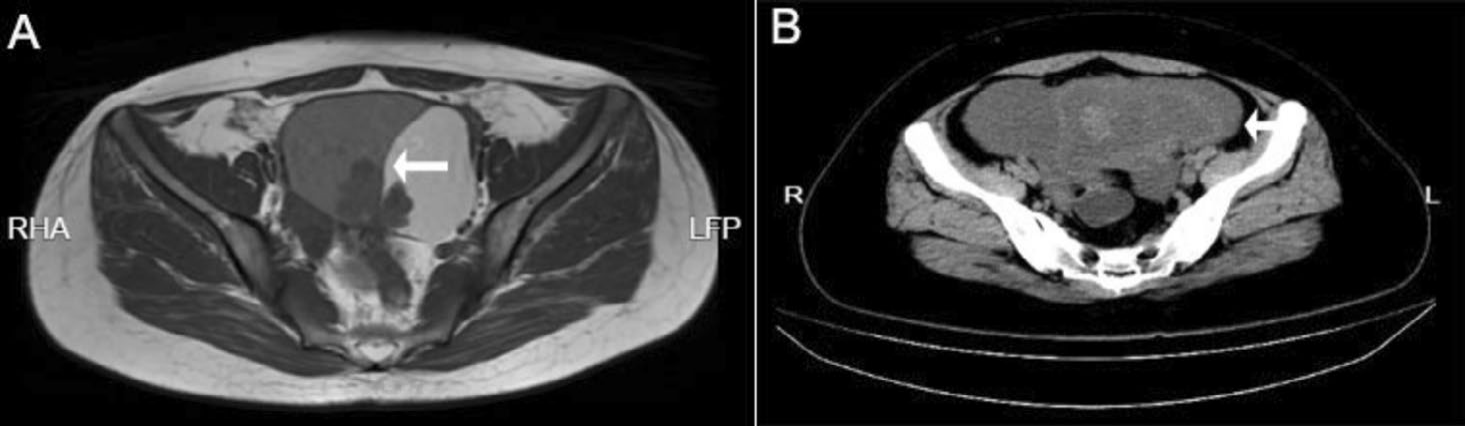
Preoperative CT results of two cases. (A) In case 1, CT imaging revealed a large cystic solid mass in the left adnexal region, measuring 106 × 65 mm, with a solid component resembling cabbage measuring 47 × 32 mm. (B) In case 2, there were short T1 and long T2 signals, presenting as a 46 × 91 mm flaky mass with a thick wall in the left adnexal area. The mass had clear edges, with a shadow measuring 25 × 29 mm.
